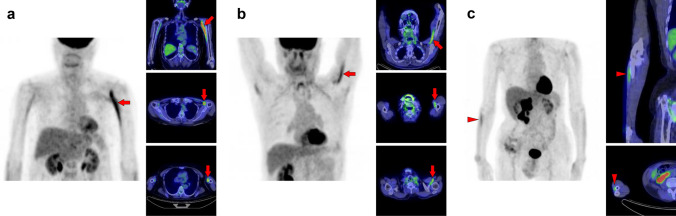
Coracobrachialis muscle and supinator muscle.* The coracobrachialis muscle can be visualized occasionally (a, b, arrows). However, untrained readers may misinterpret this linear image along the arm as retained [^18^F]FDG in vein. The supinator is also frequently visualized in [^18^F]FDG PET images (c, arrowheads). We can often observe other muscles in the arm in various forms, while it is not easy to simplify
